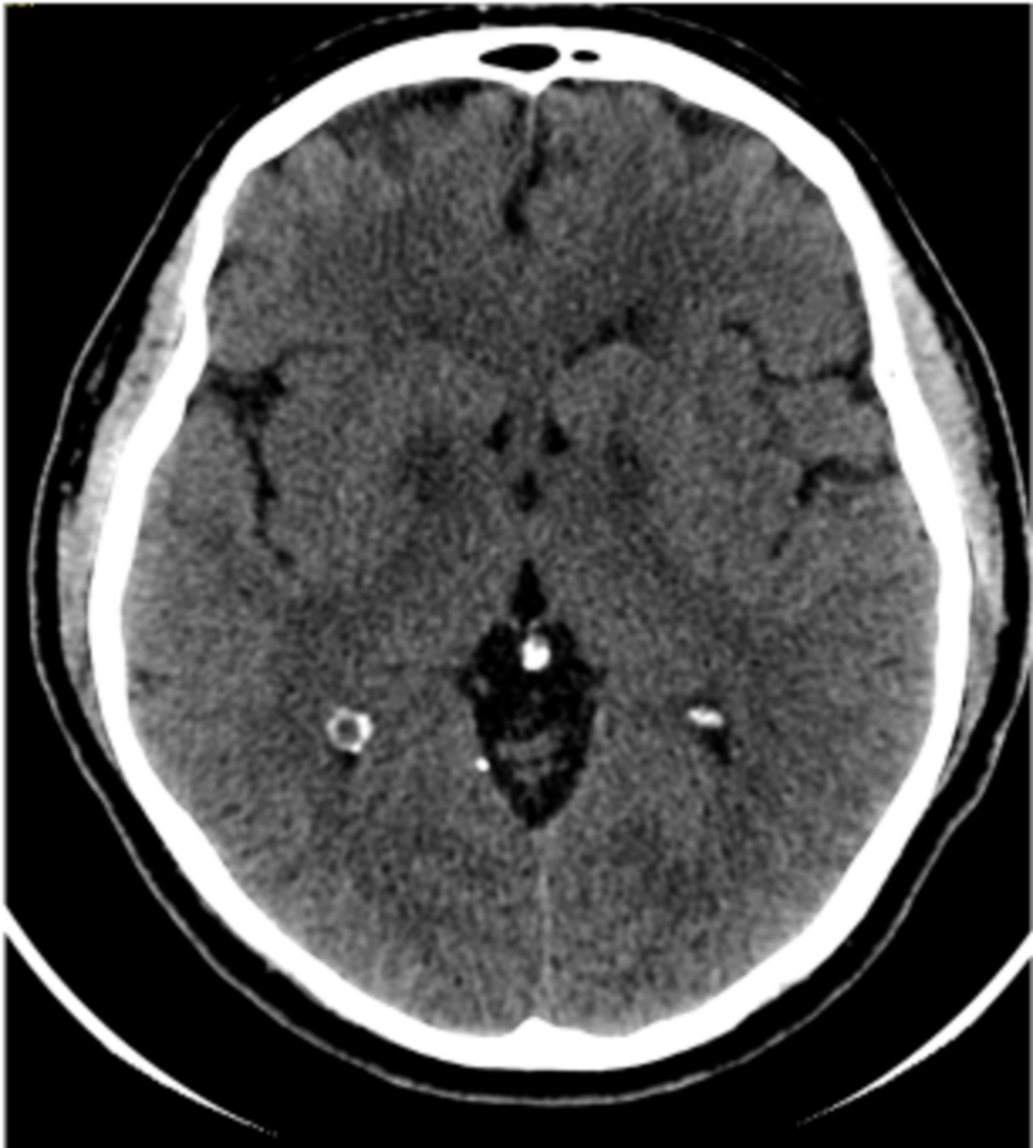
Computed tomography (CT) of the brain. Normal CT of the brain.
